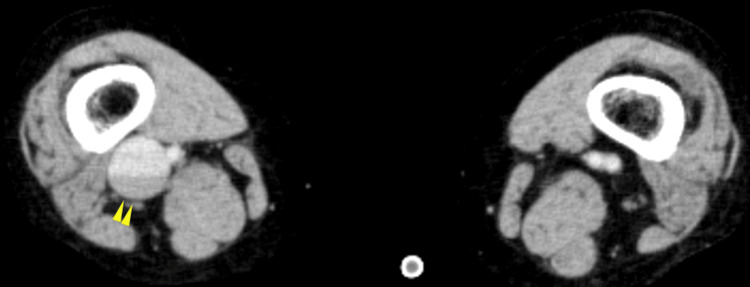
Contrast-enhanced CT revealed the presence of a right popliteal venous aneurysm (yellow arrowheads).
